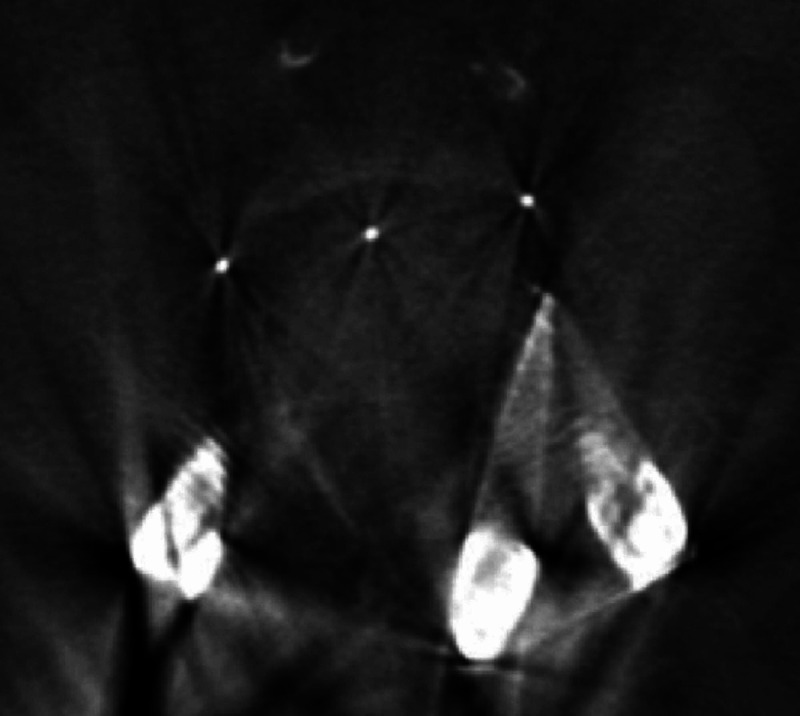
Postoperative axial computed tomography demonstrating the presence of a cortical bone trajectory screw in the same pedicle as a traditionally oriented screw
